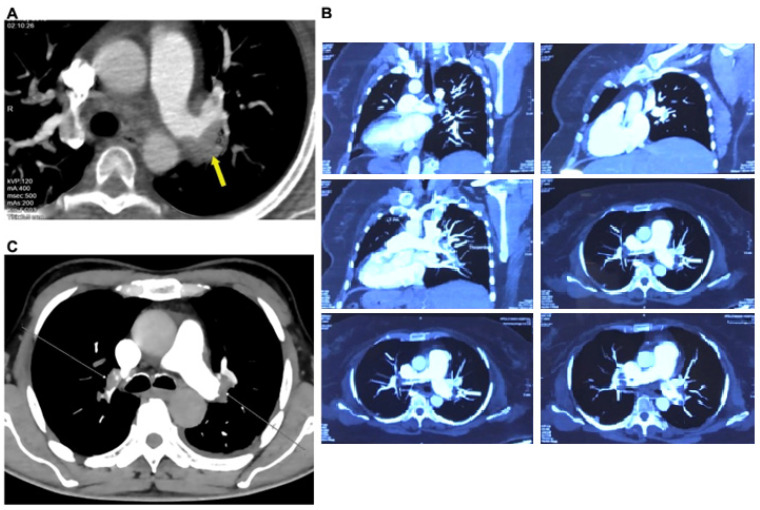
CT pulmonary angiogram in all Russell’s viper bite patients. The diagnosis of pulmonary embolism was achieved by means of CT pulmonary angiography using a contrasting dye in patients 1 (A), 2 (B), and 3 (C). The arrows indicate the thrombi. The examination was carried out on the day of admission at Manian Medical Centre.
